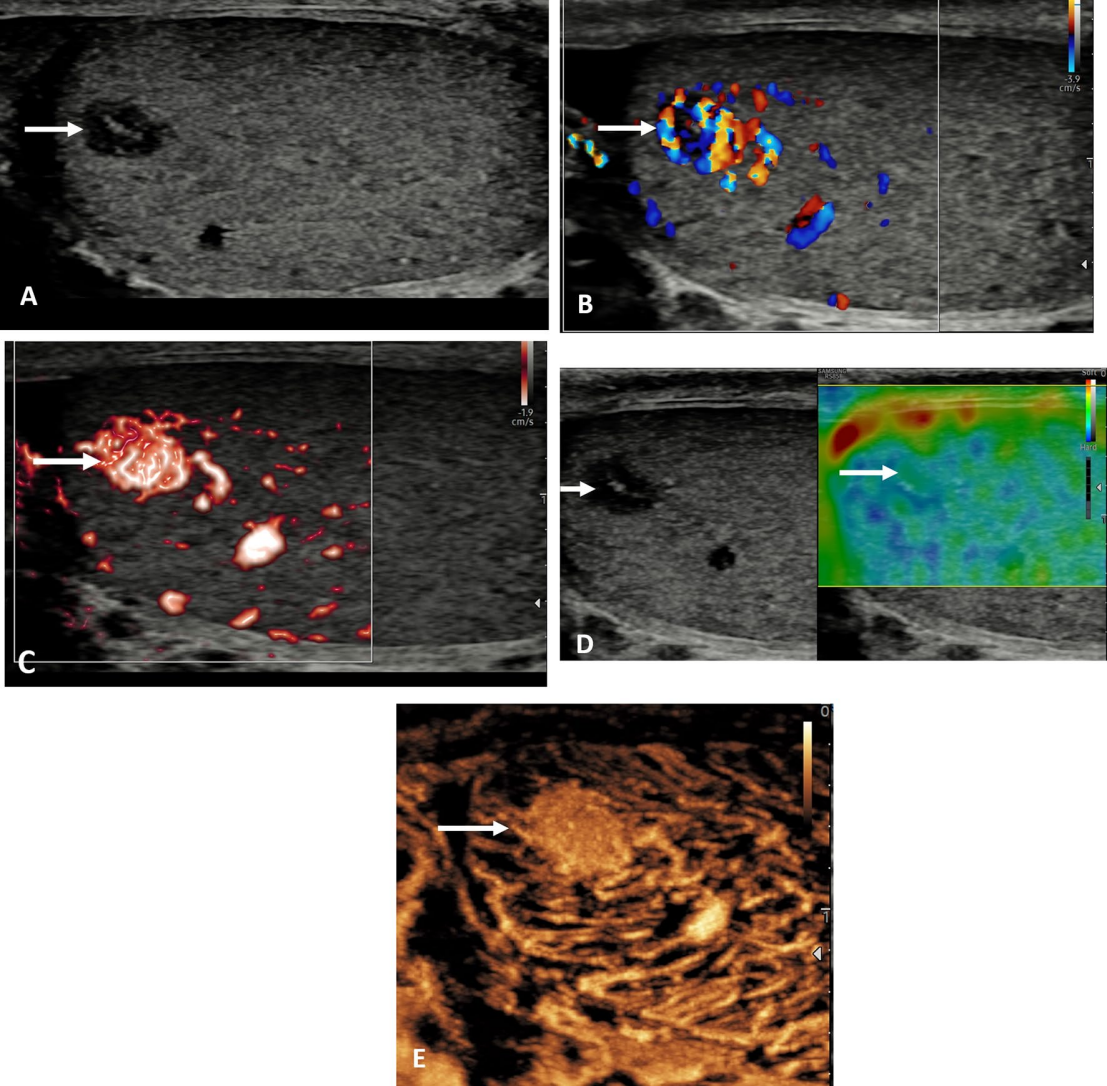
Incidental lesion in a 54 year old imaged for non-specific testicular pain. Testis sparing surgery with excision of the lesion showed Leydig cell hyperplasia. A A longitudinal image of the right testis demonstrating a 4 mm low reflective lesion (arrow). B The color Doppler image indicates increased vascularity of the focal lesion (arrow). C On the microvascular imaging there is intense vascularization present (arrow). D The tissue elastography image shows that the lesion is not stiffer than the surrounding normal tissue (arrows). E A contrast enhanced ultrasound examination, with maximum intensity projection, demonstrates a highly vascular lesion (arrow) that retains microbubble contrast at 90 s post contrast injection
